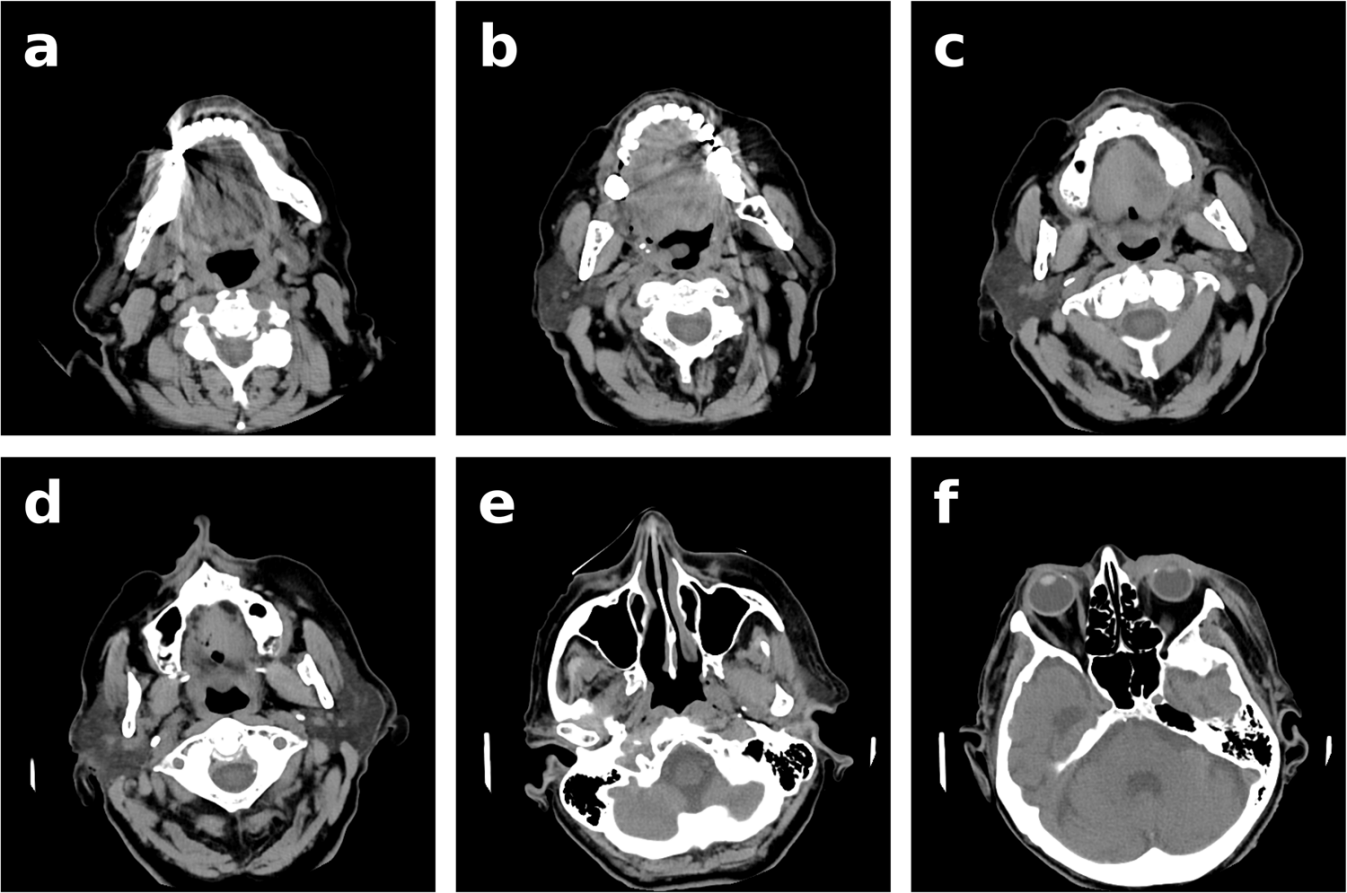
CT single slices (regions) used in the study: (a) lower row of teeth, (b) upper row of teeth, (c) end of maxilla, (d) cervical spine, (e) maximal representation of maxillary sinuses, and (f) maximal representation of eye structures
